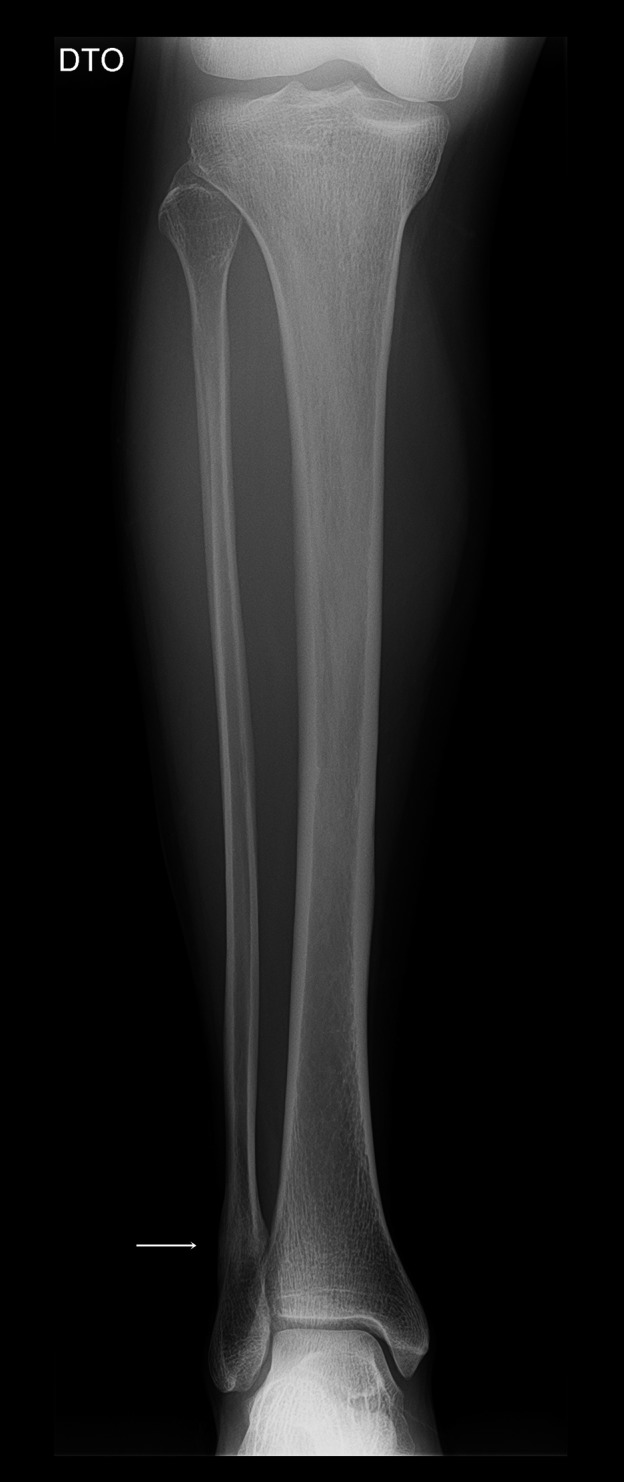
Right leg radiography, AP view, at six‐month follow‐up, confirming fracture consolidation with callus and bony remodeling (arrow).
